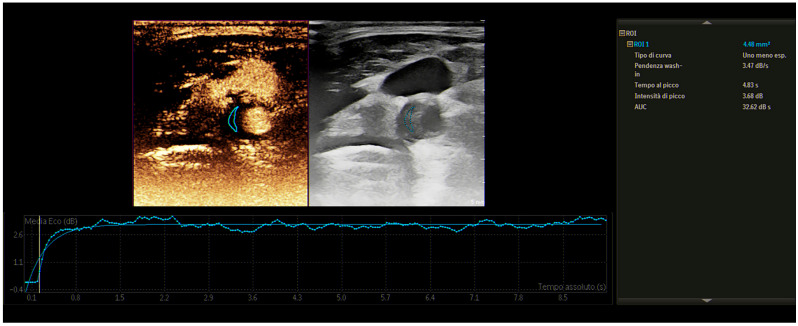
Case-based extraction of dynamic signal behavior using destruction–replenishment analysis*. Representative DR TIC analysis of an internal carotid artery plaque. Contrast-specific images acquired after operator-activated high-mechanical-index microbubble destruction show the post-flash replenishment phase. After automated motion correction, the continuous blue curve represents the mono-exponential fitted model, yielding semi-quantitative parameters including replenishment slope, time to peak, plateau intensity, and area under the curve. Analysis starts immediately after the flash; therefore, the initial signal increase reflects post-destruction microbubble refill rather than first-pass bolus transit. The curvilinear blue ROI marks the plaque region selected for TIC analysis. The oscillating blue trace represents the raw frame-by-frame contrast-enhanced ultrasound signal, dominated by microbubble backscatter and background signal fluctuations.
